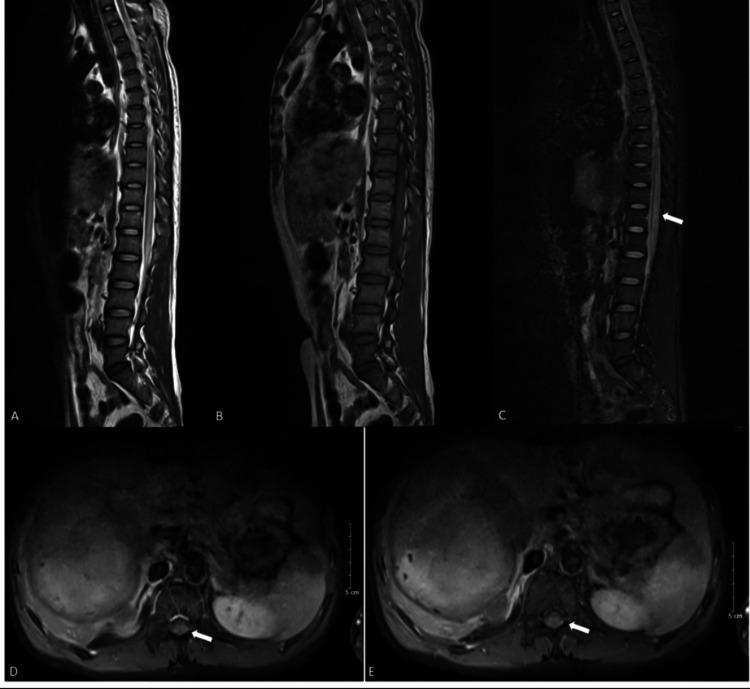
Magnetic resonance imaging (MRI) of the thoracic-lumbar spine on the next day after embolization. (A) T2-weighted image (T2W) showing hyperintense signal within the spinal cord at the T9-L1 level. (B) T1-weighted image (T1W) demonstrating hypointense signal in the same region. (C) Short tau inversion recovery (STIR) image revealing a hyperintense signal, indicating edema. (D, E) Post-contrast axial images of the corresponding spinal level showing persistent contrast enhancement of the affected region, suggesting ongoing vascularity or inflammation.
